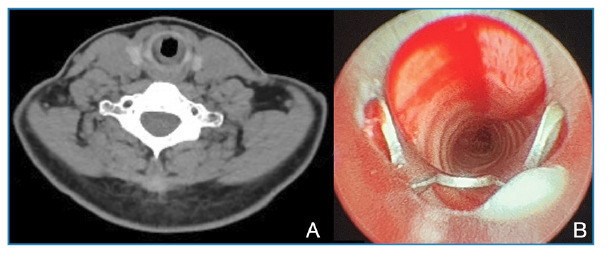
(A) Follow-up neck CT scan demonstrating complete resolution of the previously observed tracheal narrowing. (B) Follow-up rigid bronchoscopy performed after completion of treatment showing complete resolution of the subglottic tracheal narrowing with restored luminal patency.
